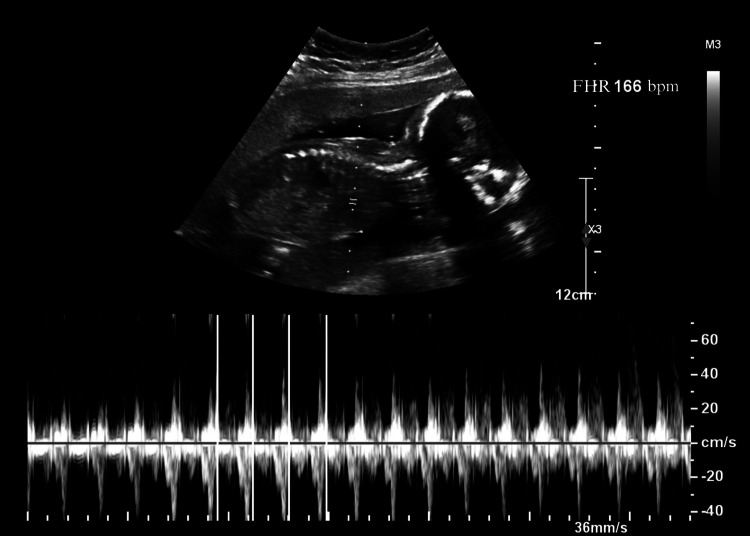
Obstetric ultrasonography Fetus in breech presentation with an FHR of 166 bpm. FHR: fetal heart rate
